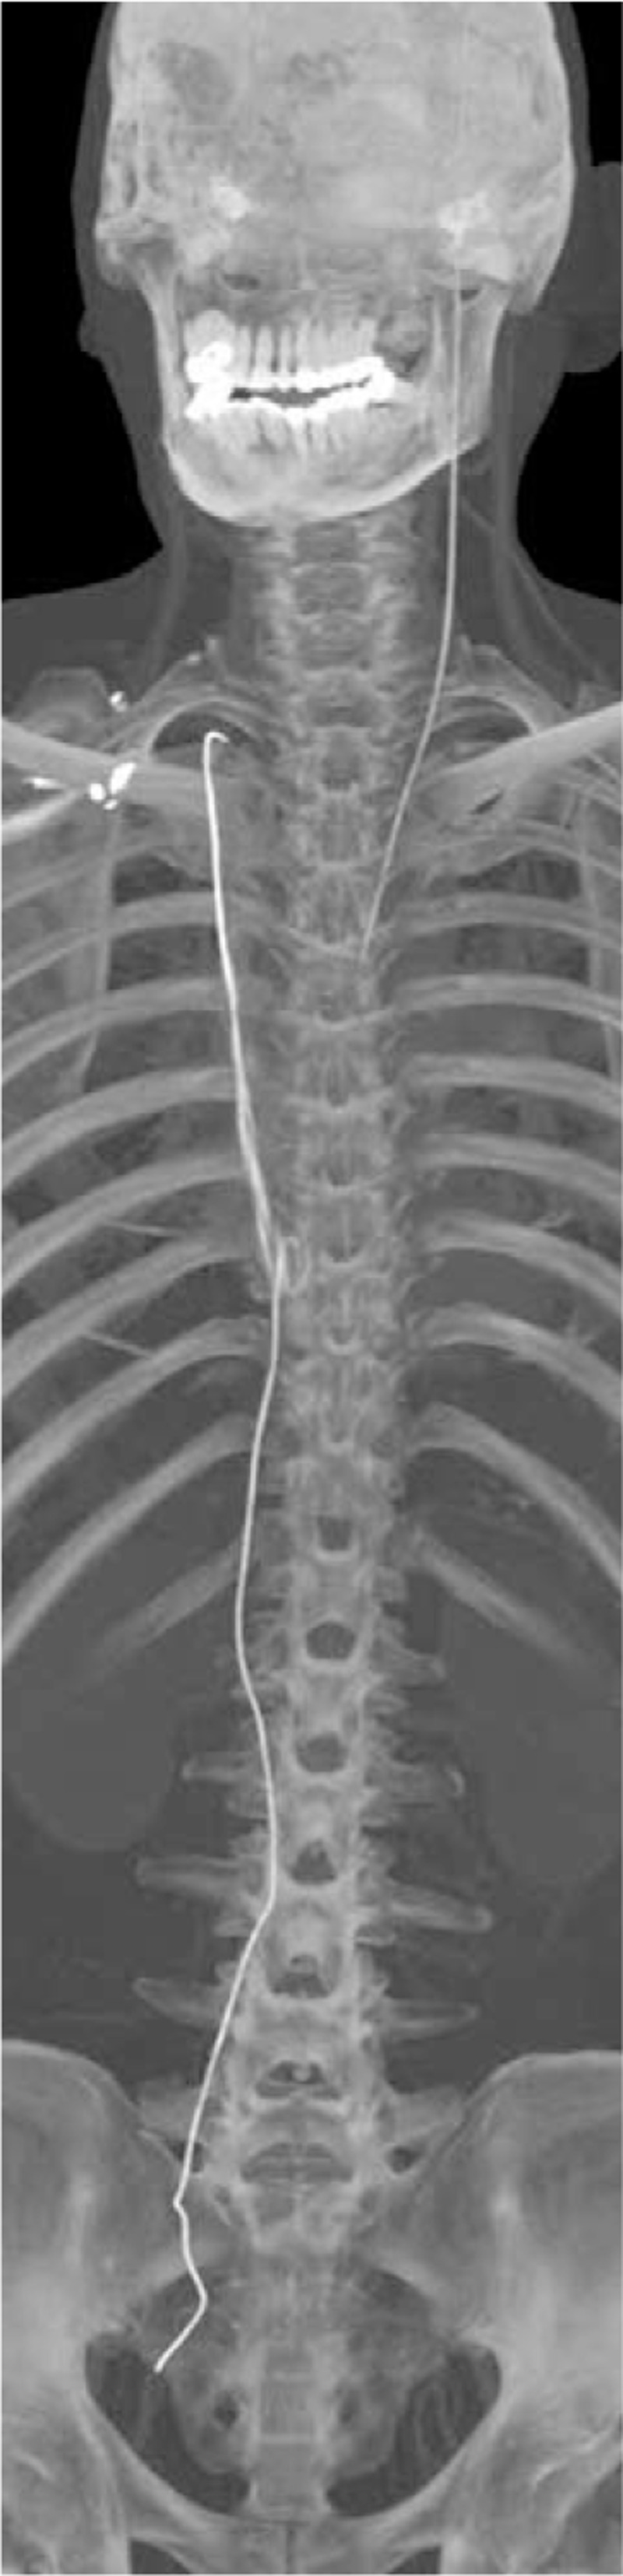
3D reconstruction of computed tomography showing one of guide wire had entered in the right brachiocephalic vena cava and the other one had entered in the cerebrum via the aortic arch.
